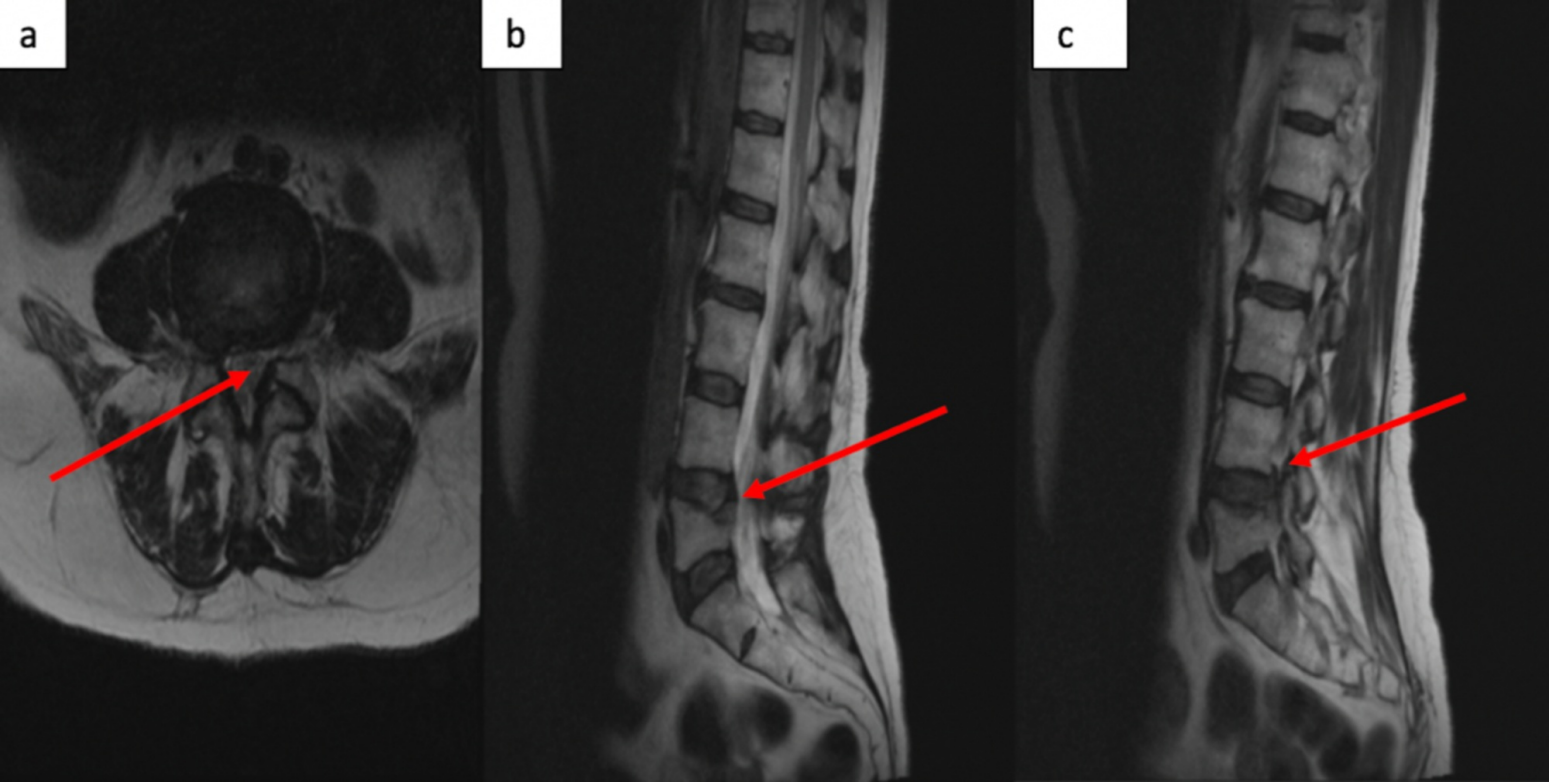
MRI of the spine T2-weighted, non-contrast MRI lumbar spine revealing central stenosis at the L4/5 level in the sagittal (b) and axial (a) views with significant thecal sac compression with severe stenosis of the traversing L5 nerve root. The lateral, sagittal view identifies moderate L4/5 foraminal stenosis with impingement on the L4 exiting nerve root (c).
